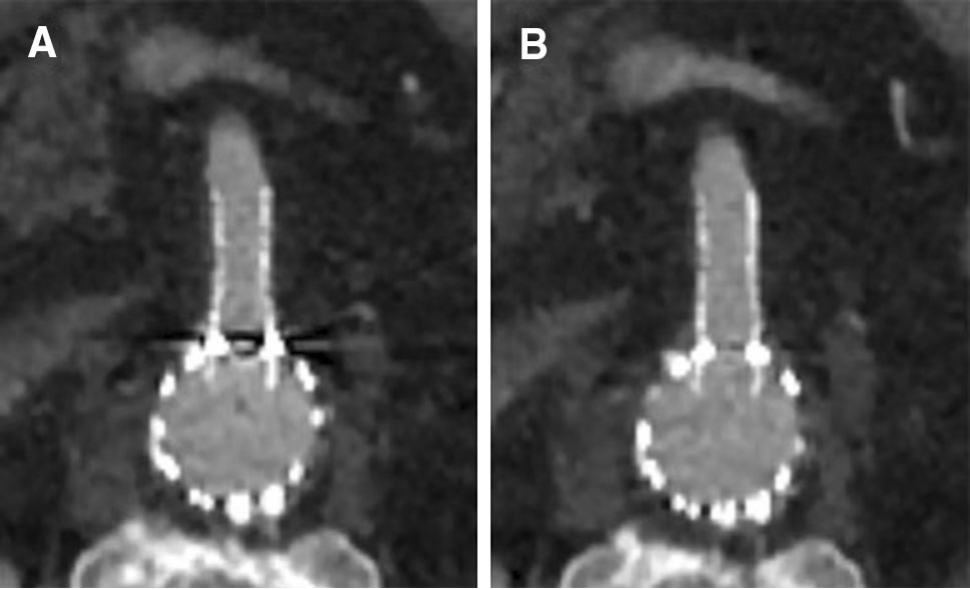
CT images (A standard (AIRD 3DE), B SEMAR) of a superior mesenteric artery in an 80-year-old male with a peripheral covered balloon-expendable stent in the fenestration. Almost complete reduction of artifacts in the SEMAR image
